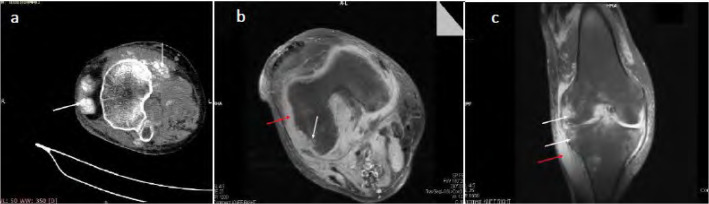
(a) CT scan of right knee (axial plane) showing extraarticular and intra-articural calcified lesions (tophi) (white arrows). (b) MRI of right knee (axial plane) showing cortical erosions at the articular surface of the lateral femoral condyle (white arrow) and a joint effusion (red arrow). (c) MRI of right knee (coronal plane) showing cortical erosions at the articular surface of the lateral femoral and tibial condyle (white arrows) and a joint effusion (red arrow).
